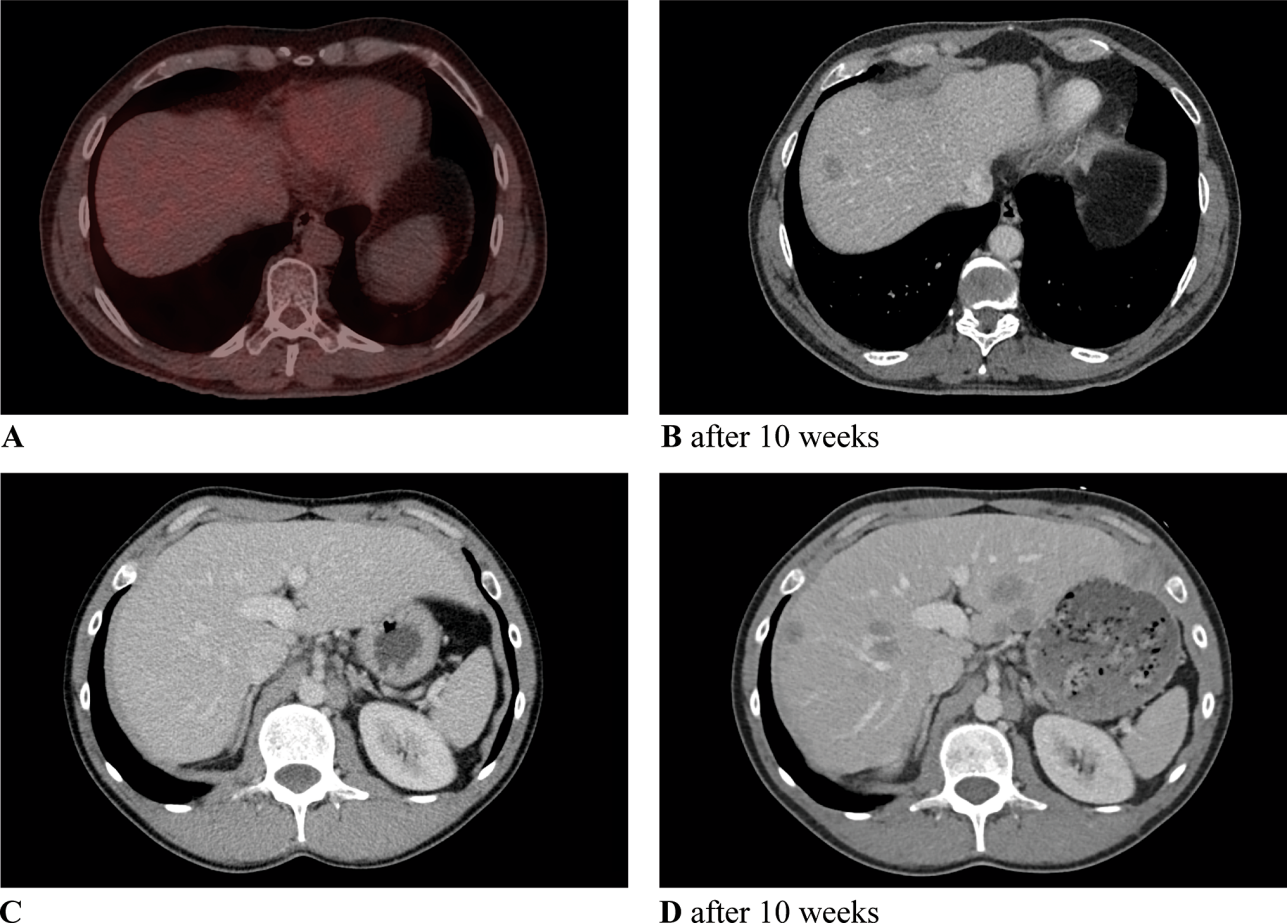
Asymptomatic recurrent melanoma during screening: A patient with pT4aN1b/stage IIIB melanoma (AJCC 7th edition)^2^ showed no metastatic disease on ^18^F-FDG PET/CT prior to radical lymph node dissection (RLND) (a), but venous-phase contrast-enhanced CT (ceCT) 10 weeks after RLND and 12 weeks after prior ^18^F-FDG PET/CT showed liver metastasis (b); A patient with pT2aN2b IIIB melanoma (AJCC 7th edition) showed no metastatic disease on ceCT (shown) and ^18^F-FDG PET/CT (not shown) prior to RLND (c), but ceCT 5 weeks after RLND and 10 weeks after prior ceCT revealed multiple liver metastases (d)
